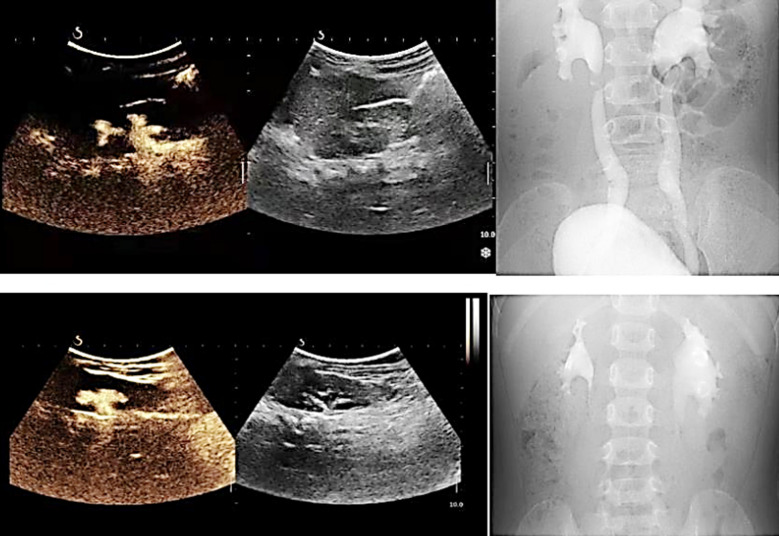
Contrast agent was observed in ureter and renal pelvis on ultrasound and abdominal x-rays respectively.
